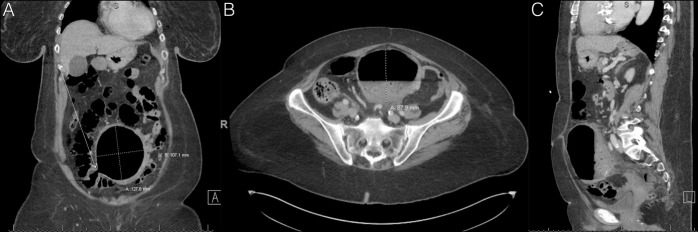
(A) Coronal, (B) transverse, and (C) sagittal view of abdominal and pelvic computed tomography with contrast showing a sigmoid colon with a giant diverticulum.
